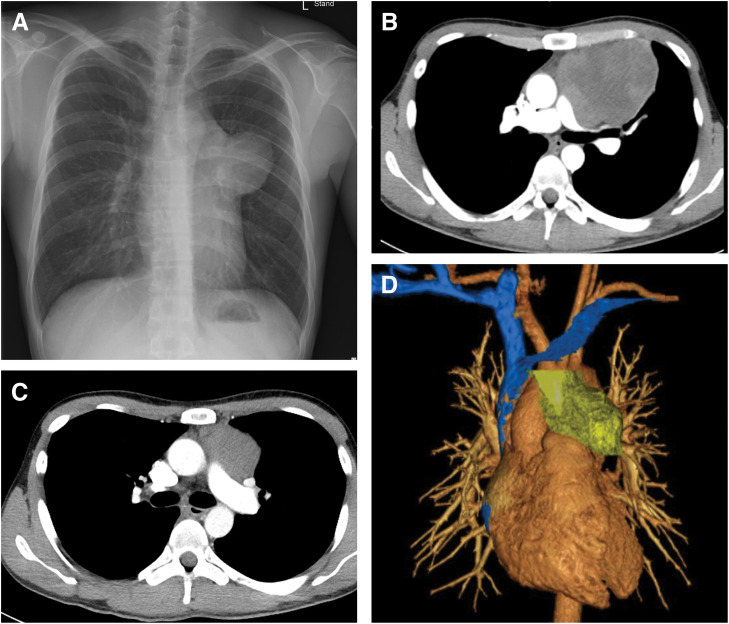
(A) Initial chest X-ray showing an opacity projecting into the left thoracic cavity. (B) Chest CT before chemotherapy showing a large mass in the anterior mediastinum. (C) Chest CT after chemotherapy showing that the mass had shrunk. (D) 3D reconstruction image before surgery.
